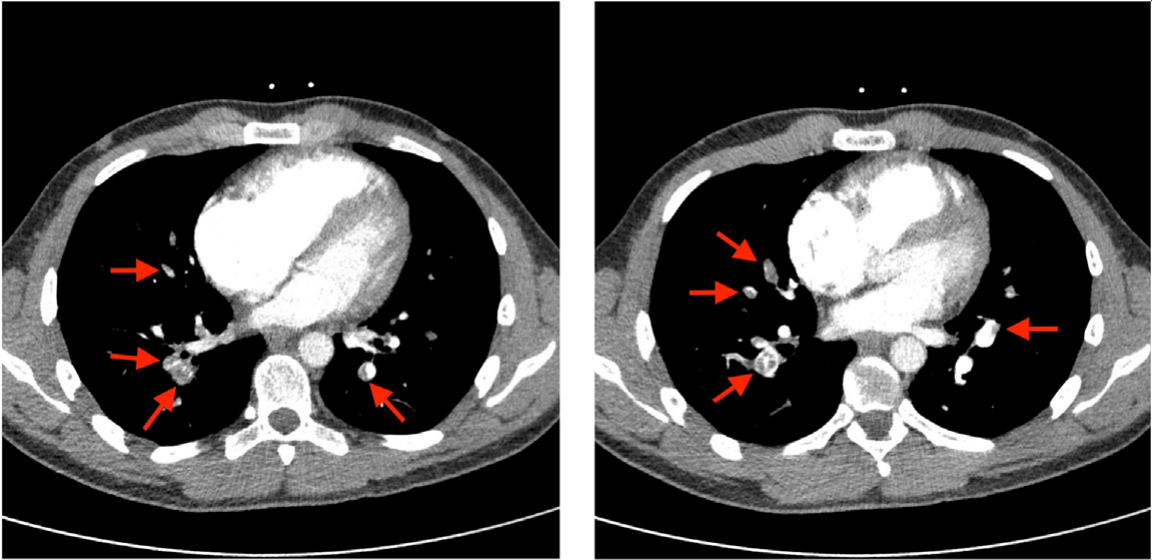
Axial contrast-enhanced Computed Tomography scan reporting bilateral pulmonary emboli.
